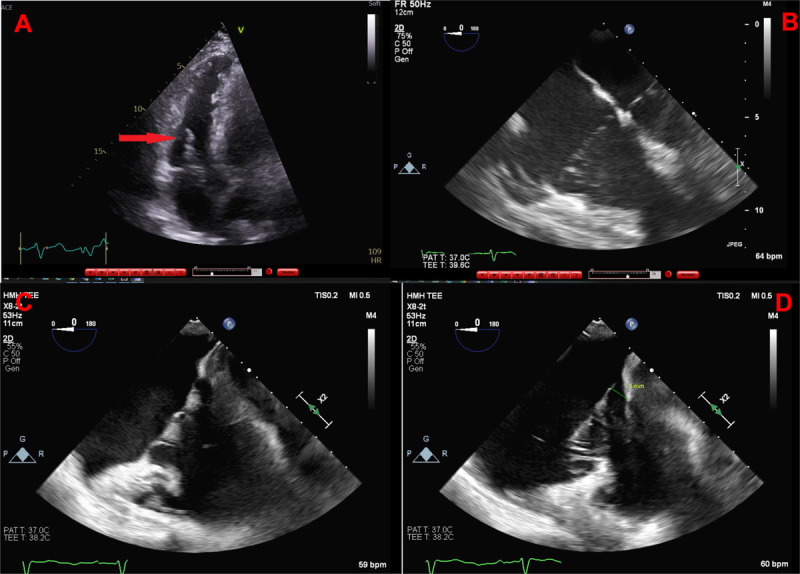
(A) Transthoracic echocardiography image demonstrates an apparent echo density on the tricuspid valve. (B) Transesophageal echocardiography (TEE) image demonstrates tricuspid prolapse with the mitral and tricuspid valves at equal level in systole. (C) TEE image shows the tricuspid leaflets in early systole at the level of the annulus. (D) TEE image shows the tricuspid leaflets in late systole prolapsing 8 mm into the right atrium.
